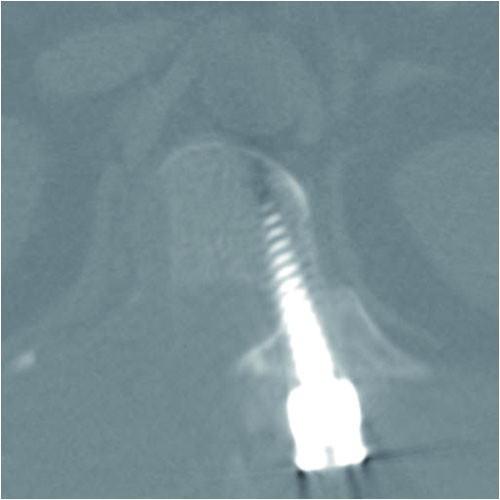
Radiographs at 1-year follow-up. Axial CT scan at the level of the T12 pedicle showing complete bony fusion and no evidence of recurrence.
